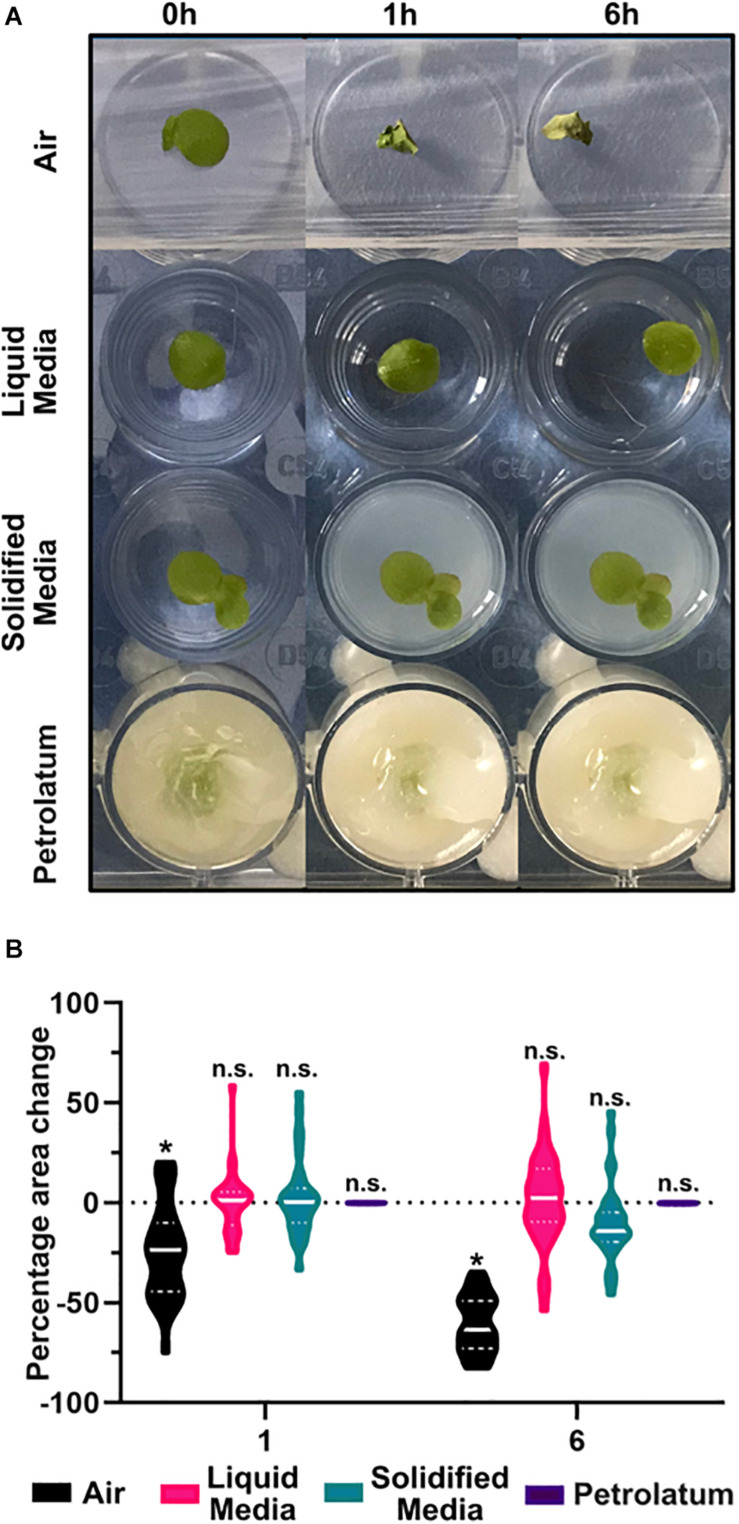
Preparation of samples in petrolatum provides the most stable method for CT imaging. (A) Representative images of *Spirodela polyrhiza* condition under different preparation methods over time. (B) Percentage change in frond area after 1 and 6 h under different preparation methods, * significant difference in mean area from start point *p* < 0.05, n.s. = No Significance in mean area change from start, *n* = 32 ANOVA with a Tukeys multiple comparison test.
